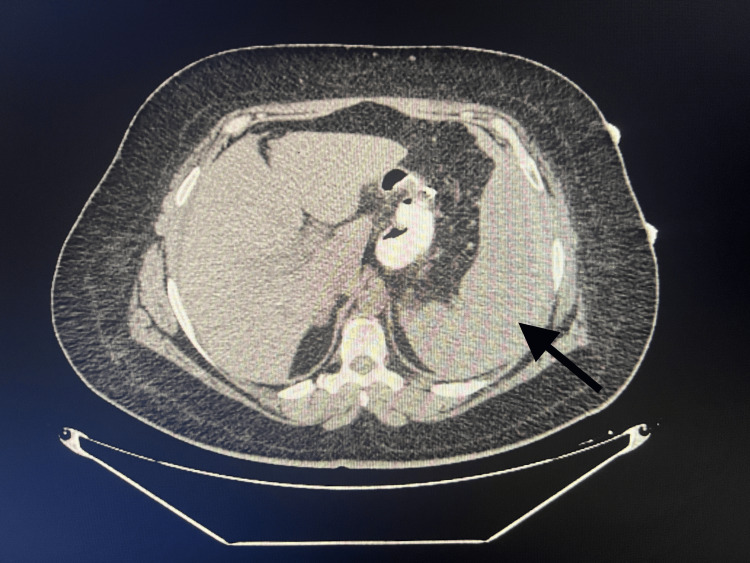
CT image of the patient on day of admission. Arrow indicates enlarged spleen.
